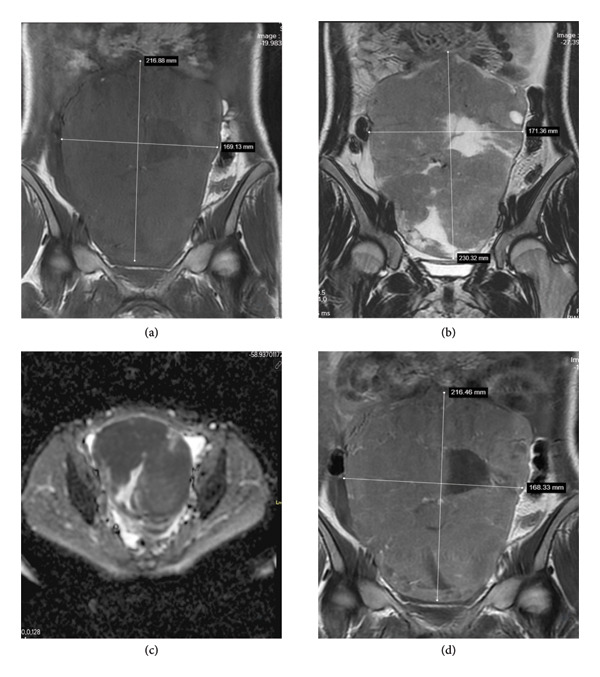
Magnetic resonance imaging (MRI) findings of the pelvic mass. (a) Coronal noncontrast T1‐weighted image demonstrates a large, heterogeneous, predominantly hypointense mass extending from the pelvic cavity to the level of the umbilicus, measuring approximately 21 × 19 cm. (b) Coronal T2‐weighted image reveals a heterogeneous mass with mixed cystic and solid components, showing variable signal intensity. (c) Diffusion‐weighted imaging (DWI) and corresponding apparent diffusion coefficient (ADC) map show restricted diffusion within the solid components of the mass, suggestive of high cellularity. (d) Postcontrast coronal T1‐weighted image demonstrates marked enhancement of the solid components.
